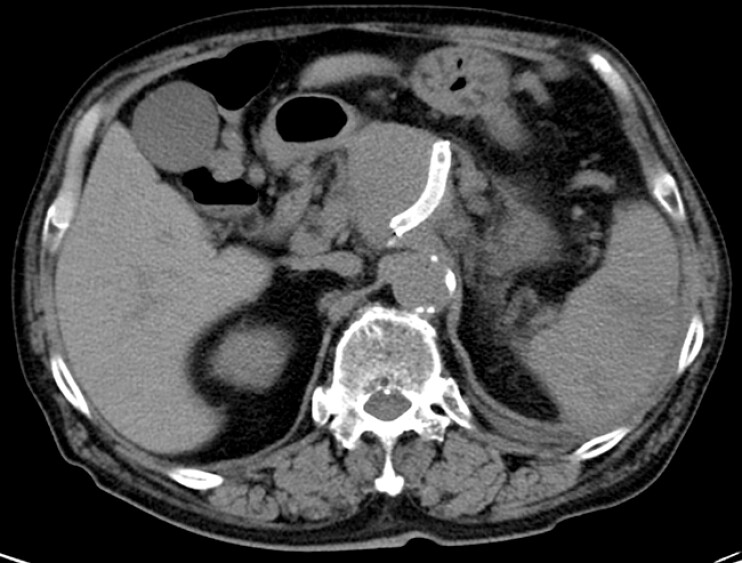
Computed tomography image showing an endovascular stent that had been placed in a splenic artery aneurysm.
